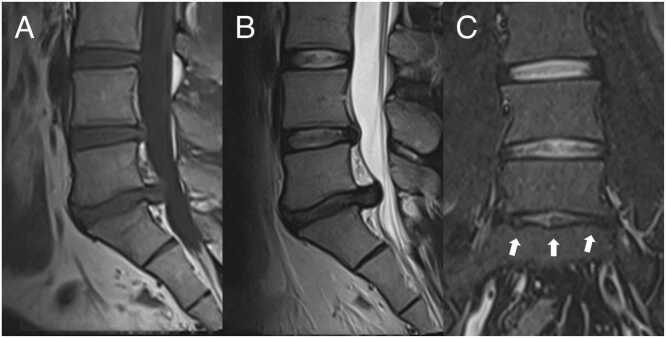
A 34-year-old patient with lumbar disc herniation at L5/S1 shown in preoperative lumbar MRI: sagittal T1-weighted (a), sagittal T2-weighted (b), and coronal STIR (c) sequences. The operated segment demonstrated a Pfirrmann grade 4 intervertebral disc. Modic type 2 changes were present in both sides of the segment. The cranial endplate showed < 25 % damage, whereas the caudal endplate exhibited ≥ 25 % damage (arrows). The combination of Pfirrmann grade ≥ 4 and ≥ 25 % endplate damage fulfilled the APD criterion.
